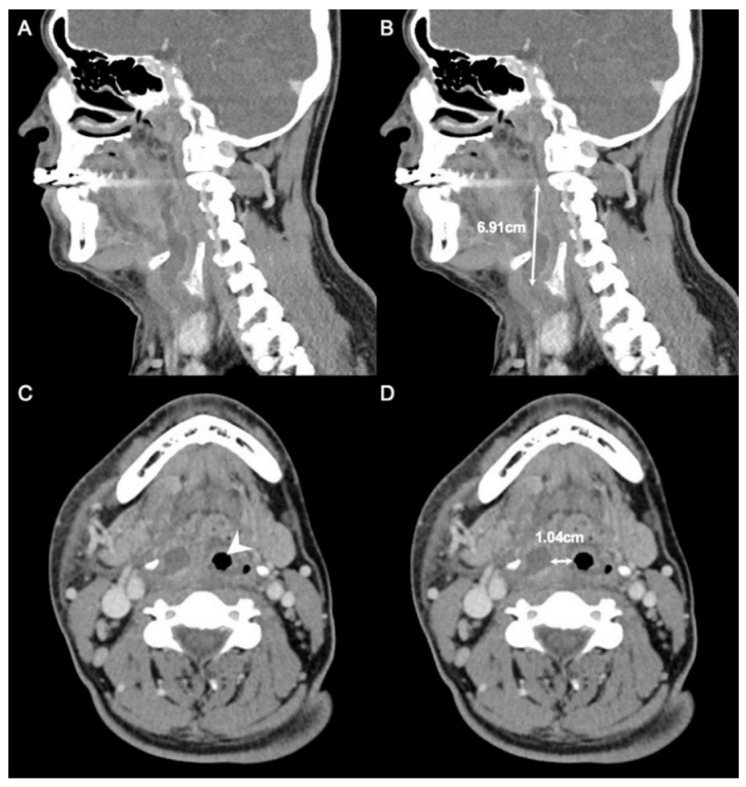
Parameters measured on computed tomography (CT) scans. (A,B) The maximum diameter of the abscess was determined based on axial, coronal, and sagittal CT scans. (C,D) The distance between the abscess and upper airway inlet was measured on axial scans. Arrowhead, upper airway inlet; double arrow, distance measured on CT scans.
